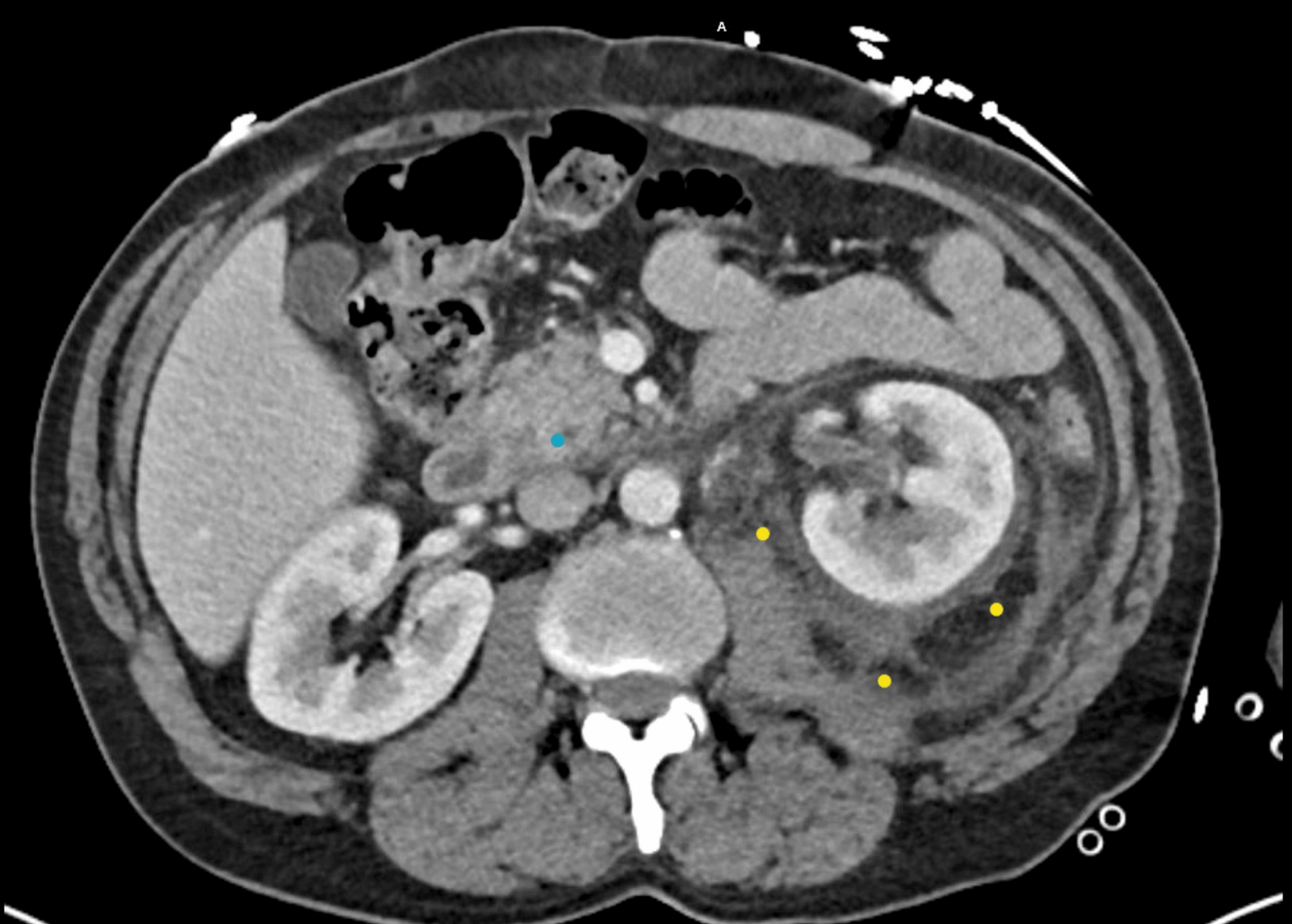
CT Abdomen Showing Perinephric Fluid Collection As Well as Peripancreatic Fluid Collection CT scan of the abdomen showing two areas of fluid collection. The yellow markings highlight the perinephric fluid collection. The blue markings highlight the peripancreatic fluid collection.
